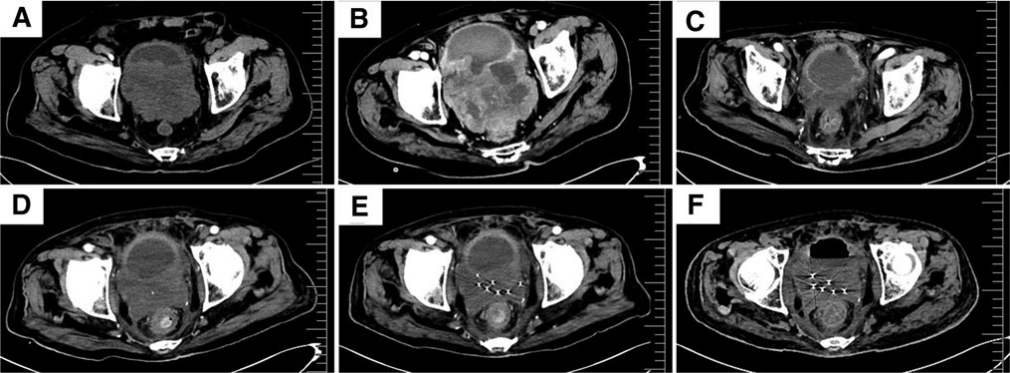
Computed tomography. (A) Obvious occupying lesion in the posterior bladder wall. (B) Obvious enlargement of the occupying lesion in the postoperative bladder wall. (C) Obvious reduction of the lesion seen after radiotherapy. (D) Disease progression 3 months after radiotherapy. (E) After particle implantation. (F) No progression after antiangiogenic therapy.
